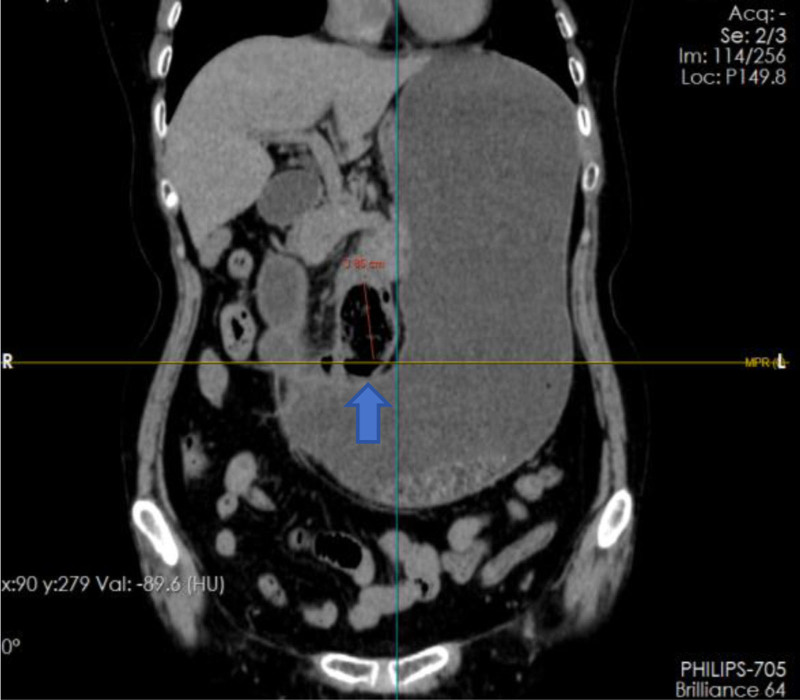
Location of the stone on abdominal CT (coronal view).
